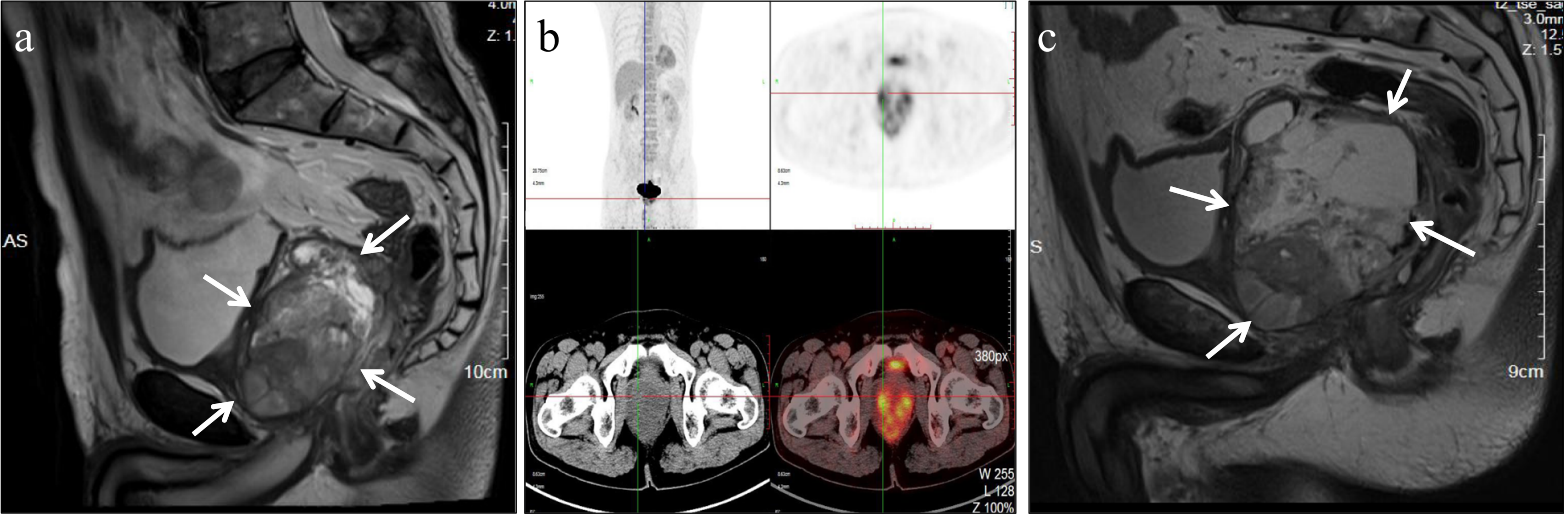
Imaging features of ARID1A deficient undifferentiated spindle cell and rhabdoid sarcoma of the prostate. (a) Magnetic resonance imaging (MRI) revealing a large, ill-defined, prostatic mass lesion with circumferential extension into the rectal wall (*as indicated by arrows*). (b) Positron emission tomography-computed tomography scan showing a mixed mass of the prostate with an uneven increase in fluorodeoxyglucose metabolism. (c) MRI showing that the prostatic tumor is significantly enlarged with central areas of hemorrhage, necrosis and cystic change (*as indicated by arrows*), after 2 months of chemotherapy with etoposide and cisplatin
